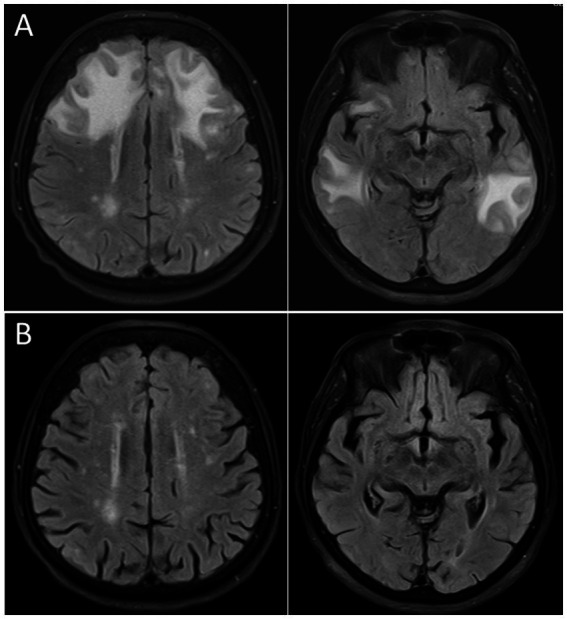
Cranial magnetic resonance imaging (cMRI), fluid attenuated inversion recovery (FLAIR) sequence, of an 87 years old woman presenting with severe delirium upon *Escherichia coli* urosepsis. (A) Distinct vasogenic edema in the frontal and temporal lobe compatible with a posterior reversible encephalopathy syndrome (PRES) 2 days after onset of clinical symptoms. (B) Follow-up imaging 5 weeks later demonstrating complete resolution of the vasogenic edema.
